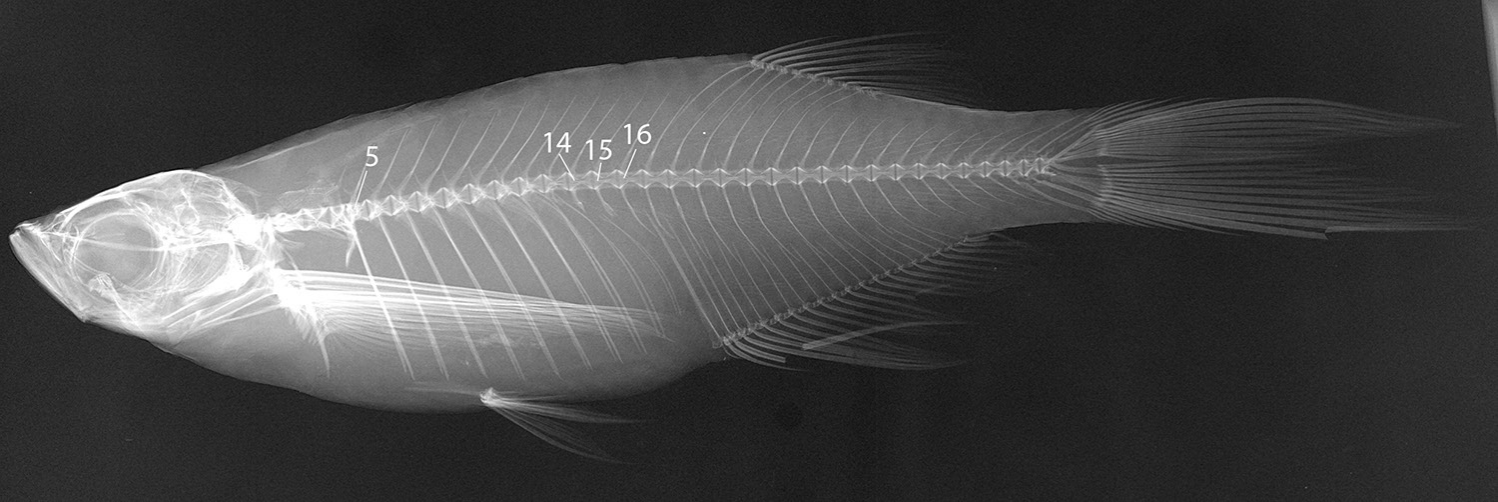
X-radiograph of *Laubuka
